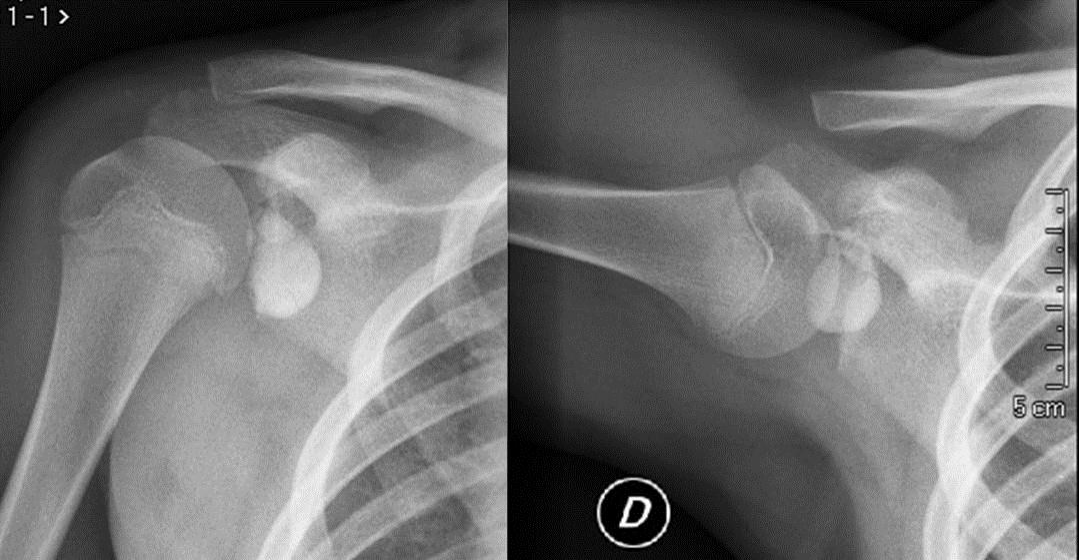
Calcific tendinopathy (CT) of the subscapularis tendon in a 13-years-old boy.
